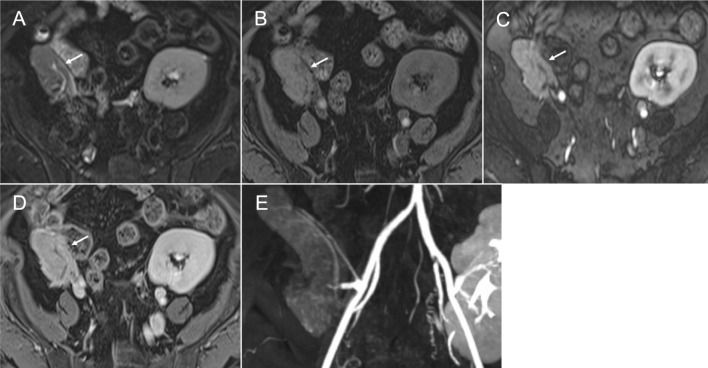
Normal findings on magnetic resonance imaging. A normal pancreas graft (arrow) demonstrates mildly high signal intensity on fat-suppressed (FS) T2-weighted imaging (A) and high signal intensity on FS T1-weighted imaging (B). Contrast-enhanced sequences show homogeneous enhancement on the arterial phase (C) and delay phase (D). Magnetic resonance angiography shows the course and smooth vascular contours of the transplant vasculature (E)
